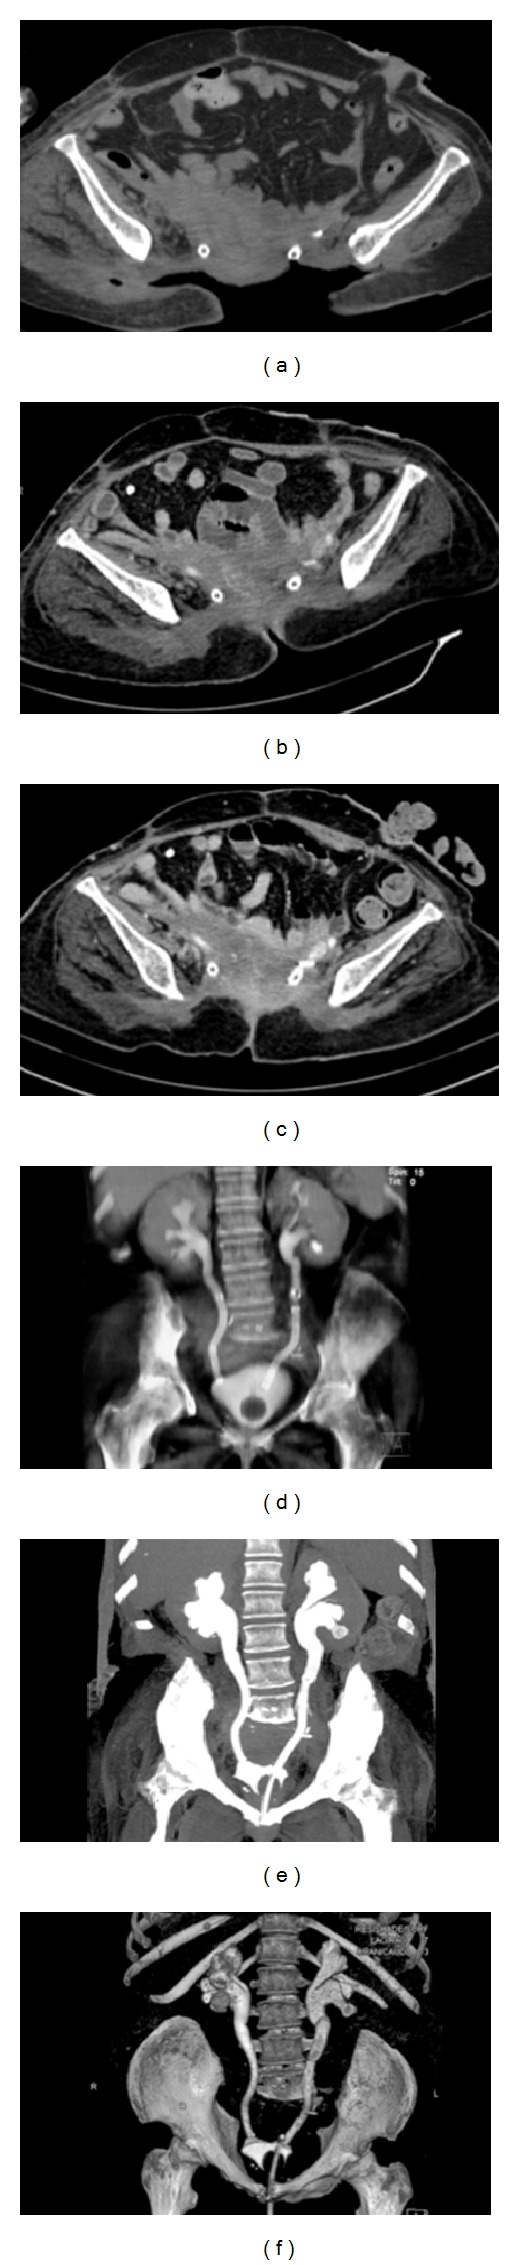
Contrast-enhanced CT scan (a) the day after the ureteral stents were positioned. Contrast-enhanced CT scan performed in January 2012 (b) and in April 2012 (c): the surgical wound shows a progressive healing and there is no ureteral leakage. Maximum intensity projection (MIP) CT-reconstruction showing the correct position of the two ureteral stents after 8 (d) and 18 months from the procedure (e). 3D CT-volume rendering after 18 months from the procedure (f).
